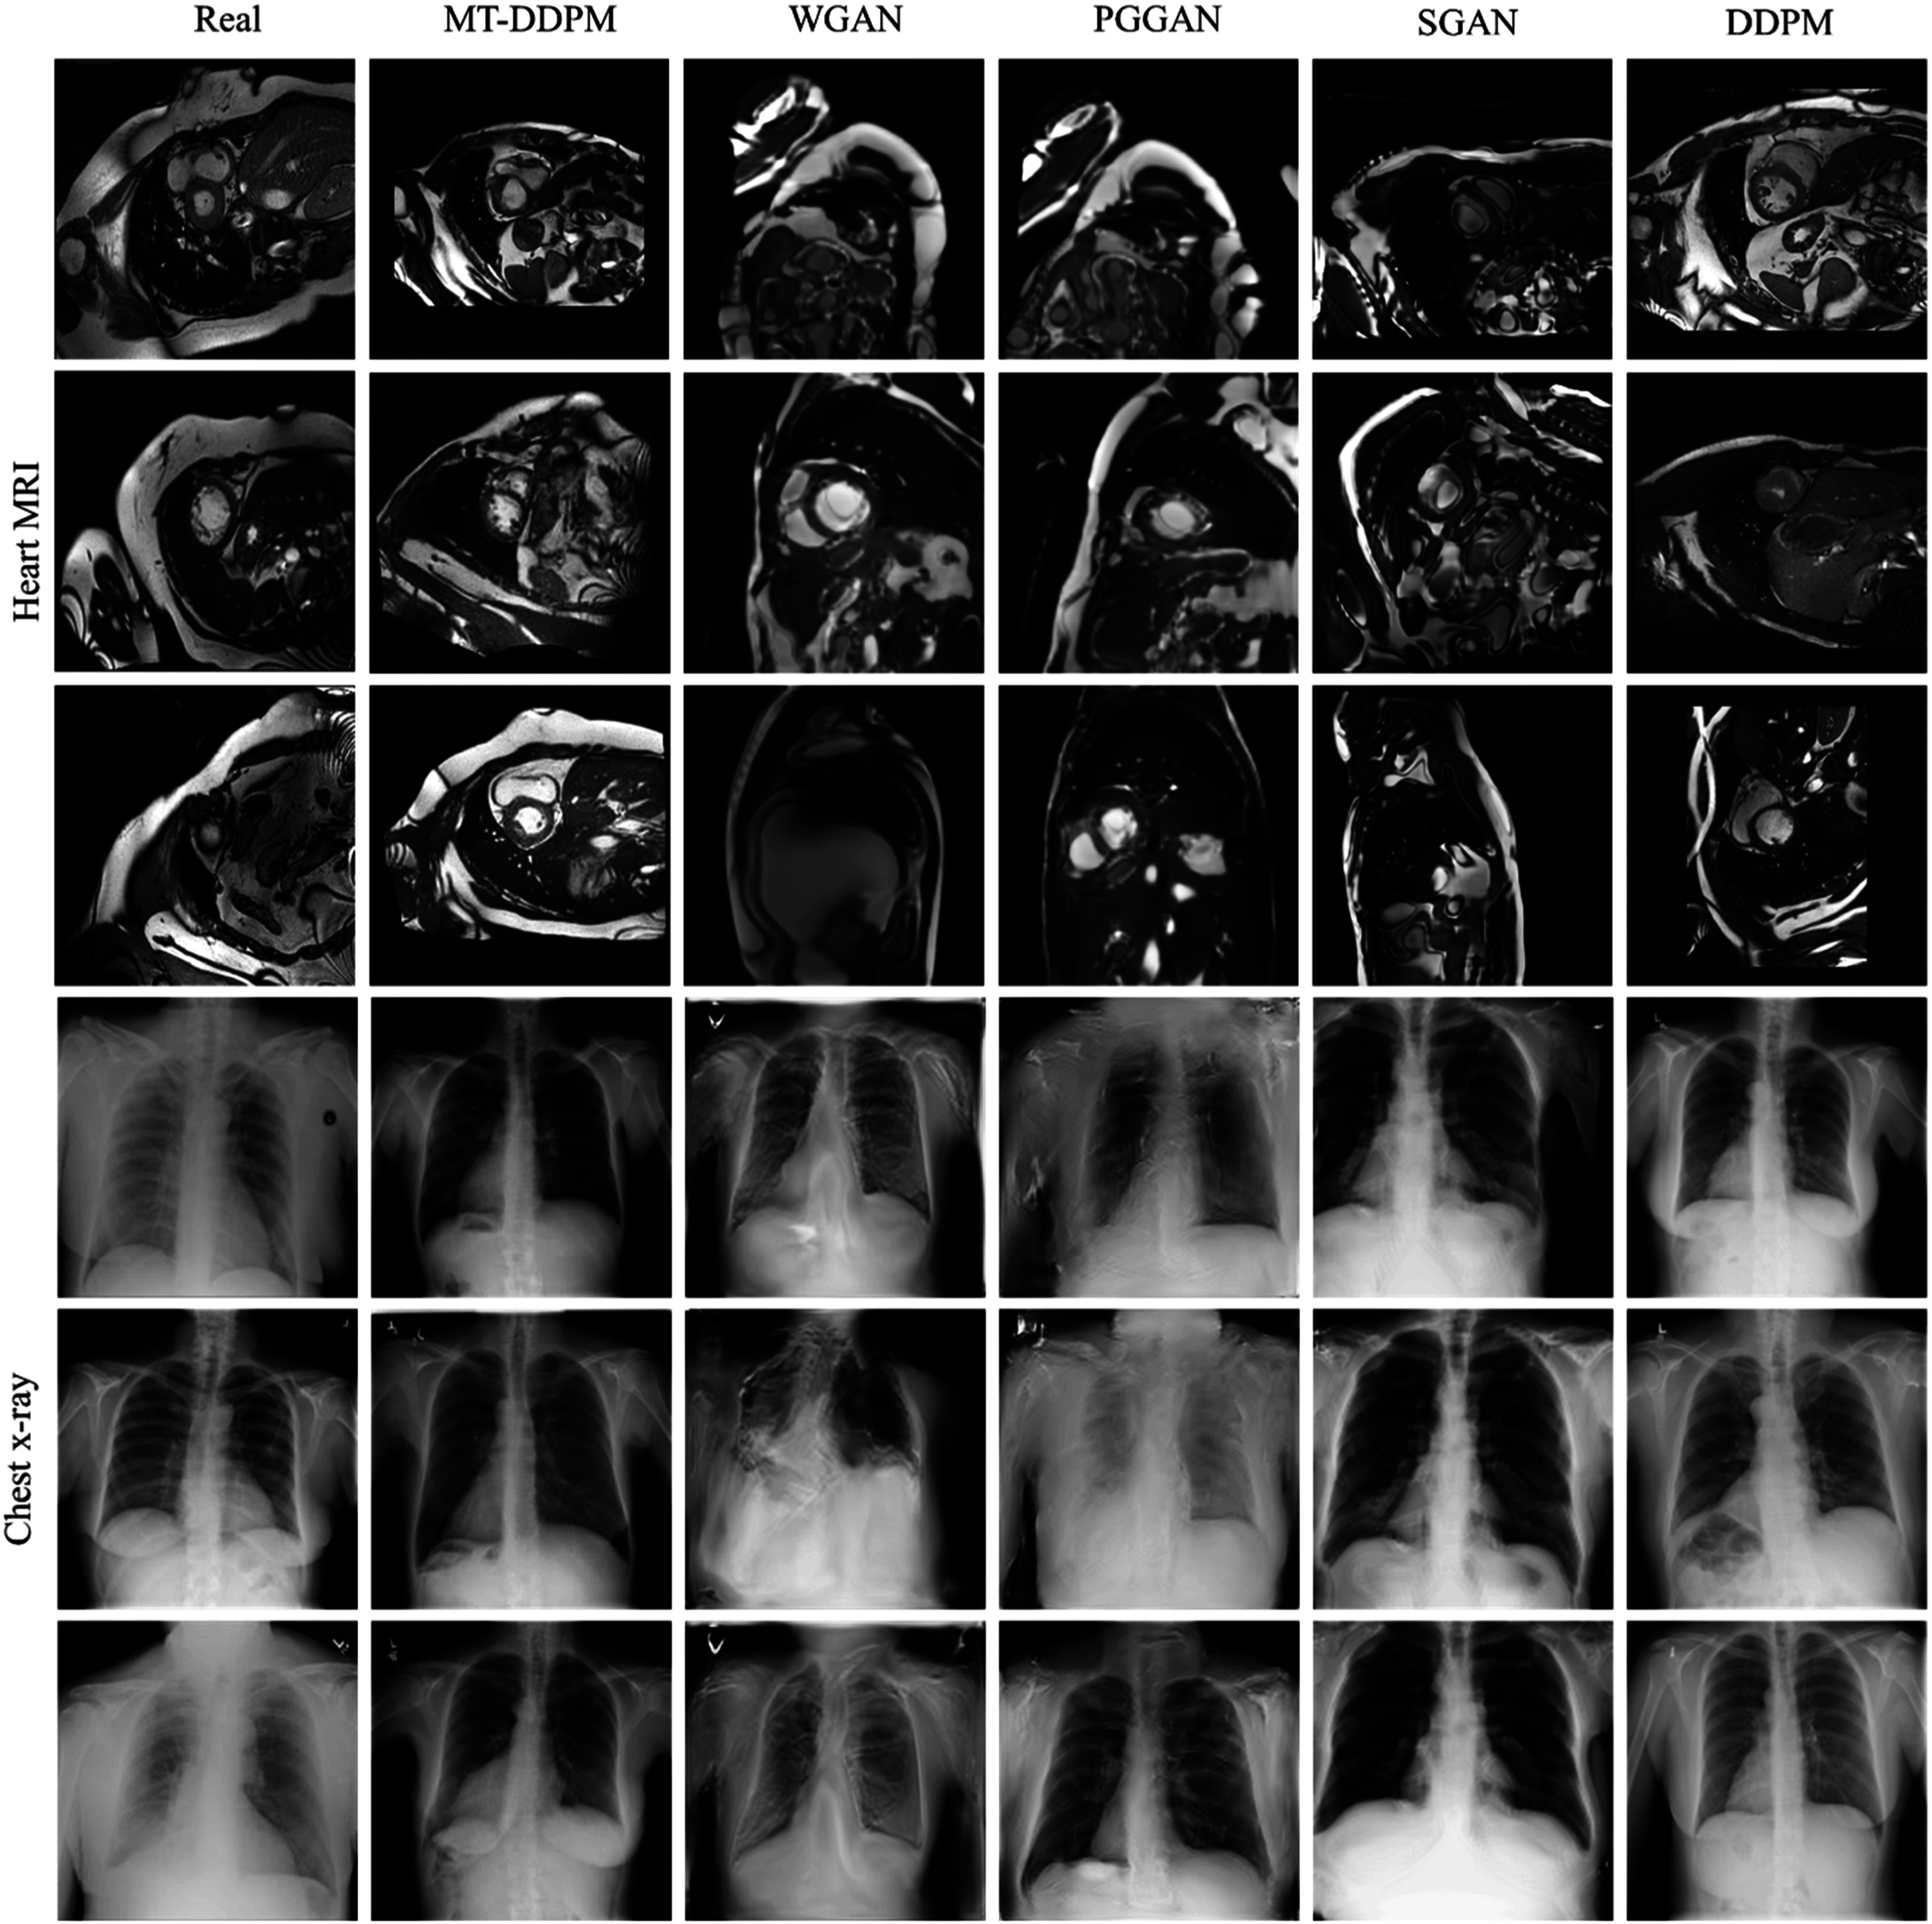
Synthetic and real images from chest x-rays, heart MRI. In each dataset, the real images, the synthetic images of the proposed MT-DDPM, WGAN, PGGAN, SGAN, and DDPM are presented in column-wise. Three slices of chest x-rays (row #1 to #3), heart MRIs (row #4 to #6) are displayed. Notice that for visualization purposes, the examples from different modalities were resized to the same resolution but could with different pixel spacings.
